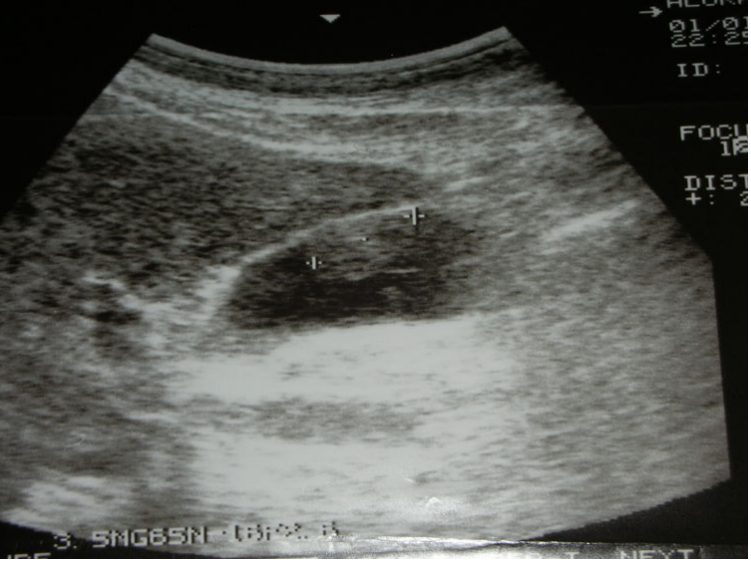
Ultrasound evidence of metastatic melanoma of the gallbladder.
